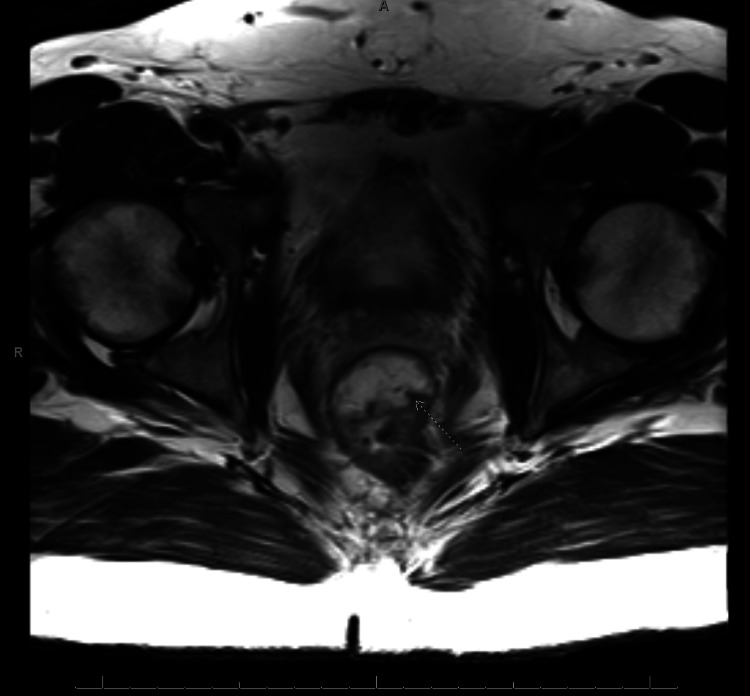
Axial T2 weighted MRI of the spaceOAR (hypointense) with arrow indicating disruption of the anterior rectal wall.
